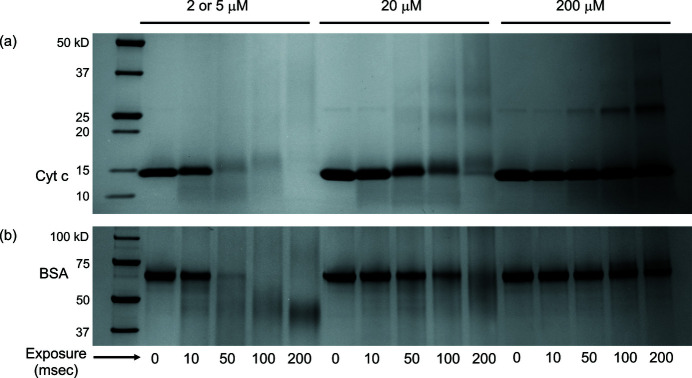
Concentration dependence of X-ray damage. SDS-PAGE of cyt *c* (*a*) and BSA (*b*) after X-ray irradiation of 0–200 ms and for different protein concentrations. BSA concentrations were 2, 20, and 200 µ*M*, while cyt *c* concentrations were 5, 20, and 200 µ*M*. For reference, the calculated absorbed radiation dose by the buffer was 162 Gy for a 10 ms X-ray exposure and 3240 Gy for a 200 ms exposure.
